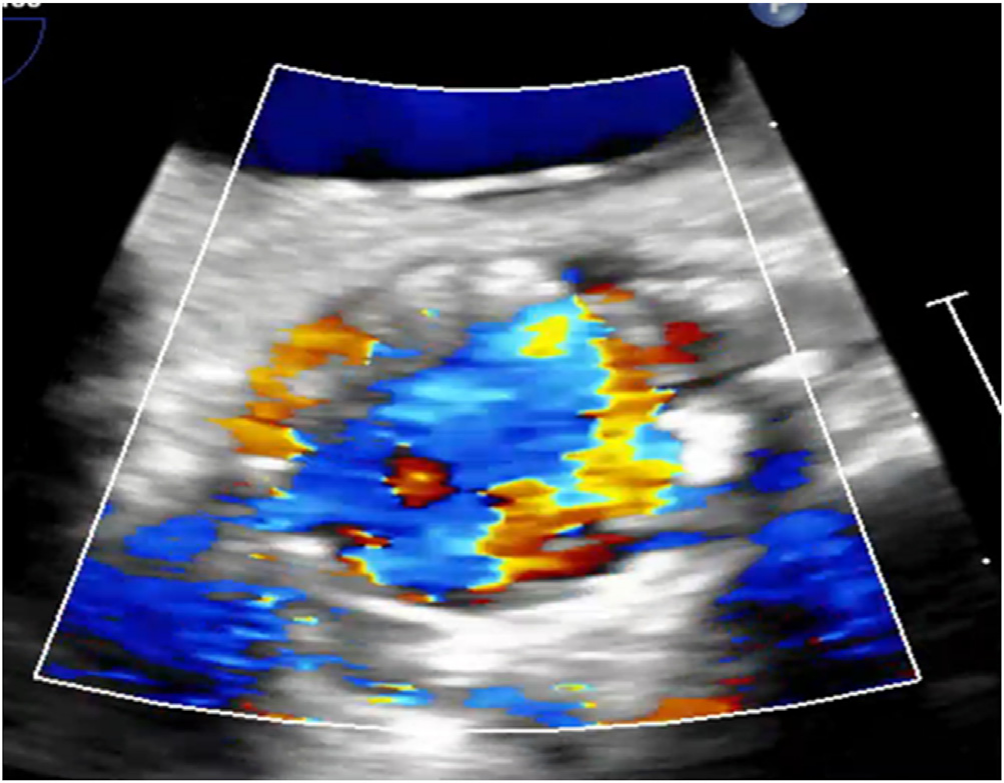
Transesophageal echocardiogram short axis of aortic valve view showing frozen leaflet causing severe AR. Abbreviation: AR, aortic regurgitation.
